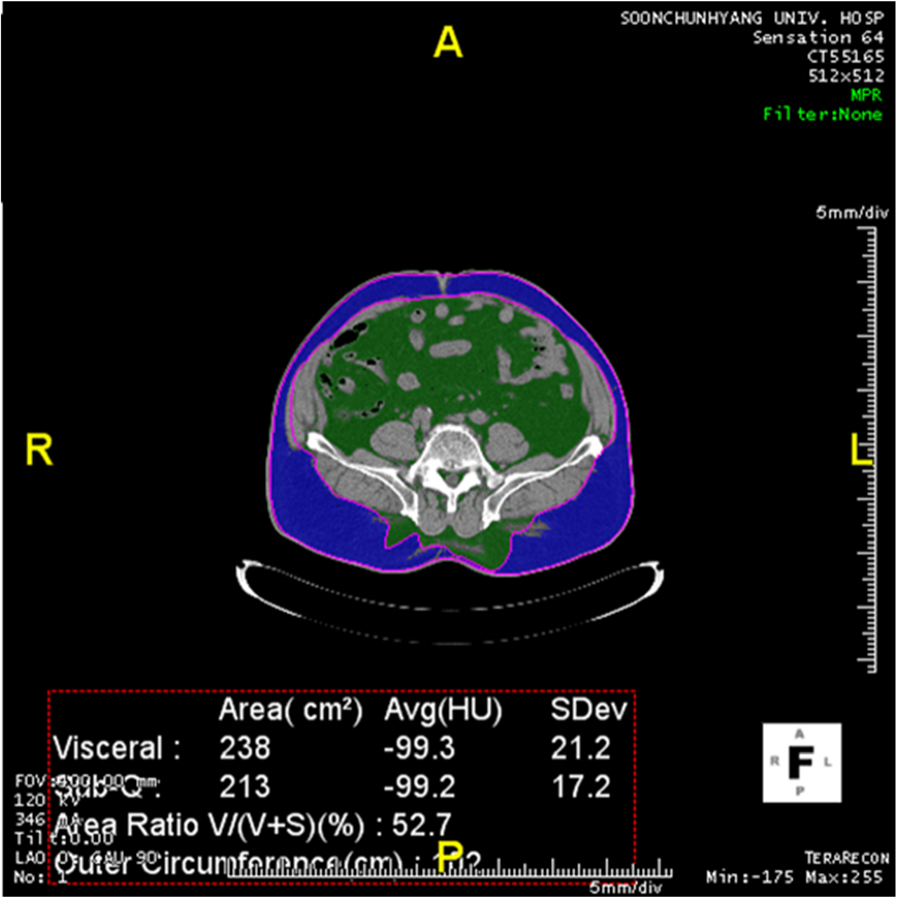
CT fat delineation in a male with obesity and visceral obesity whose visceral fat was 238 cm
